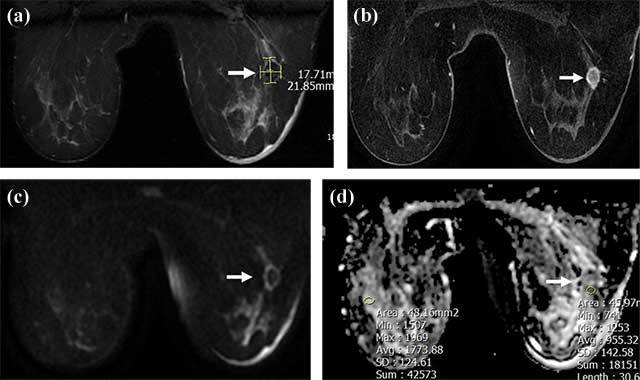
A 18 × 22 mm irregular mass lesion in the right breast lower-outer quadrant, hypointense (arrow) in T2-weighted images (a) with rim enhancement (arrow) in contrast images (b), showing diffusion restriction (arrow) in DWI; ADC and rADC values are 0.955 and 0.544, respectively (c, d). Tru-cut biopsy results diagnosed invasive ductal carcinoma.
